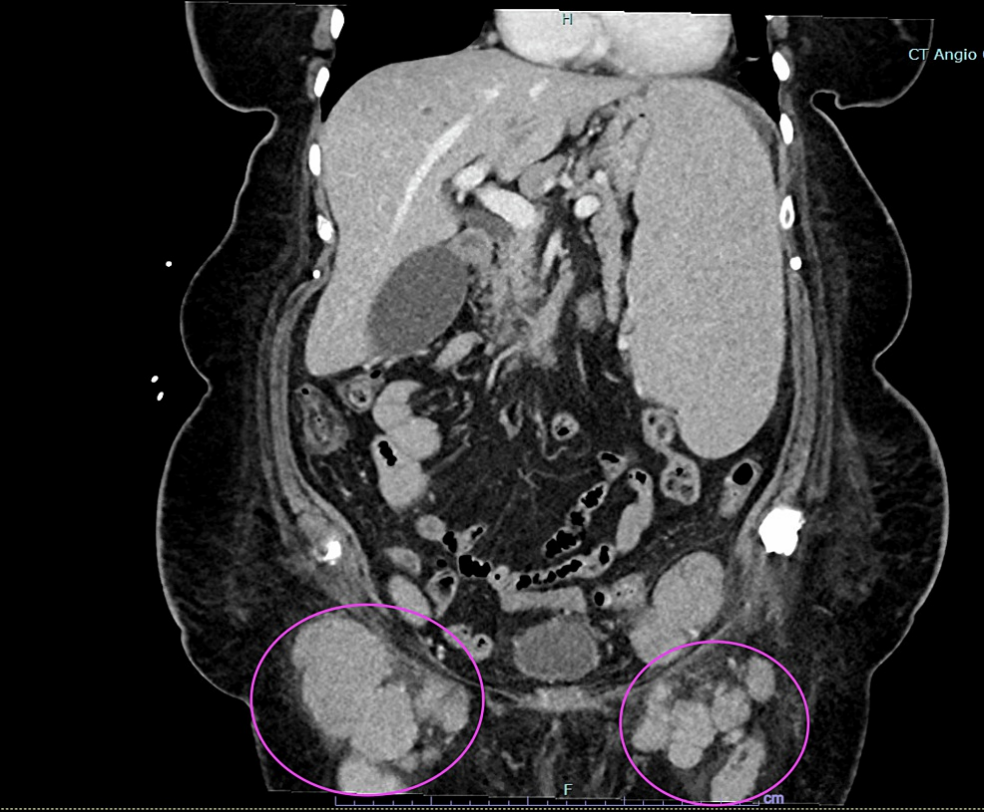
Bilateral inguinal lymphadenopathy on CT imaging (circled in purple color)
